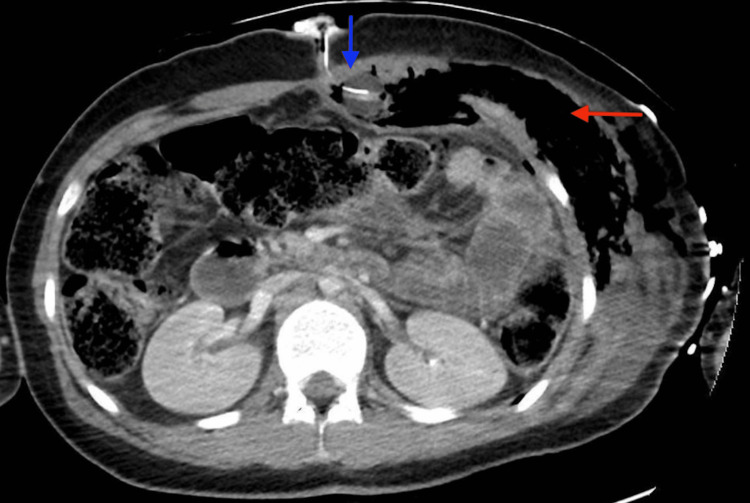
Axial contrast-enhanced CT of the abdomen demonstrating a malpositioned gastrostomy tube. The tube tip is located within the retrorectus space (blue arrow), with associated extraluminal enteral contents tracking within the anterior abdominal wall (red arrow), consistent with gastrostomy tube displacement and leakage.
